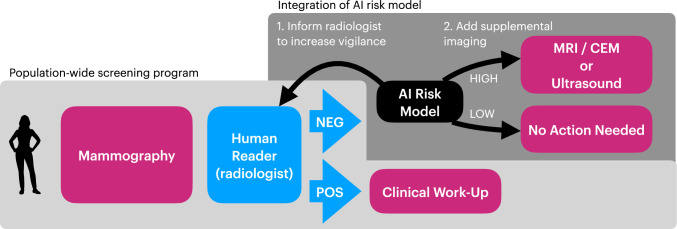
The integration of an AI risk model to inform the human reader of the standard screening modality (mammography) and to identify women for supplemental imaging by Magnetic Resonance Imaging (MRI) or Contrast-Enhanced Mammography (CEM) or Ultrasound. If the human reader does not detect any image signs of potential cancer, i.e., the mammogram is negative, then the mammograms are further analyzed by an AI Risk model. If the model assigns a high risk of cancer, there are several options how to proceed: (i) the human reader may be informed and re-read the mammogram, (ii) the individual may be recalled for contrast-enhaned MRI, contrast-enhanced mammography, or ultrasound. If the AI Risk model assigns a low risk of cancer, no further action would be needed.
